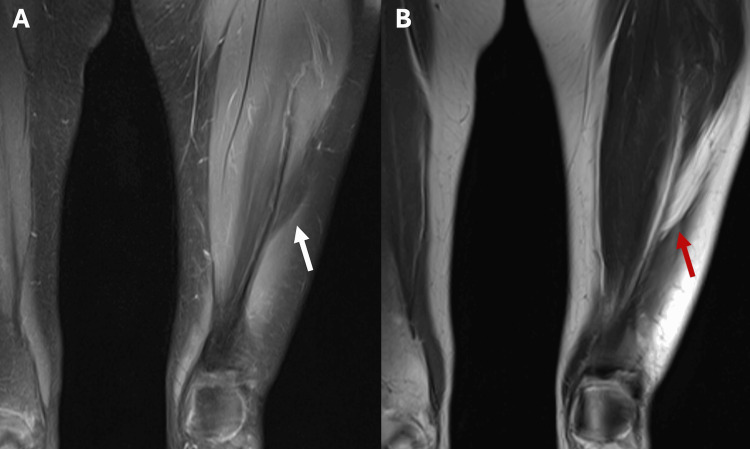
MRI images of the intramuscular lipoma within the patient's left leg (A) T2 weighted MRI showing non-enhancing mass (white arrow) within the vastus lateralis; (B) T1 fat-saturated MRI image showing homogenous isoenhancing mass (red arrow) to surrounding fat.
